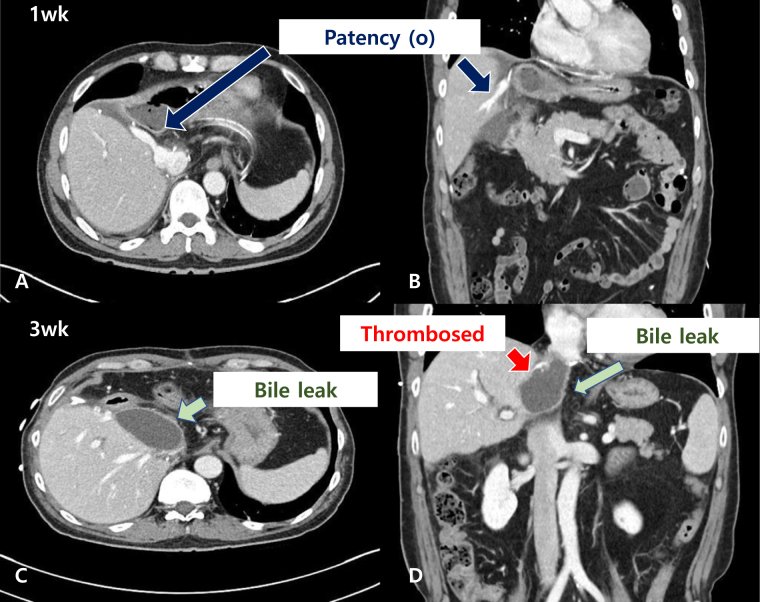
ADM graft thrombosis associated with postoperative bile leak. (A, B) CT at postoperative week 1 shows patent ADM conduit (blue arrows).(C, D) CT at postoperative week 3 demonstrates complete thrombosis (red arrows) adjacent to a large biloma (green arrows).
